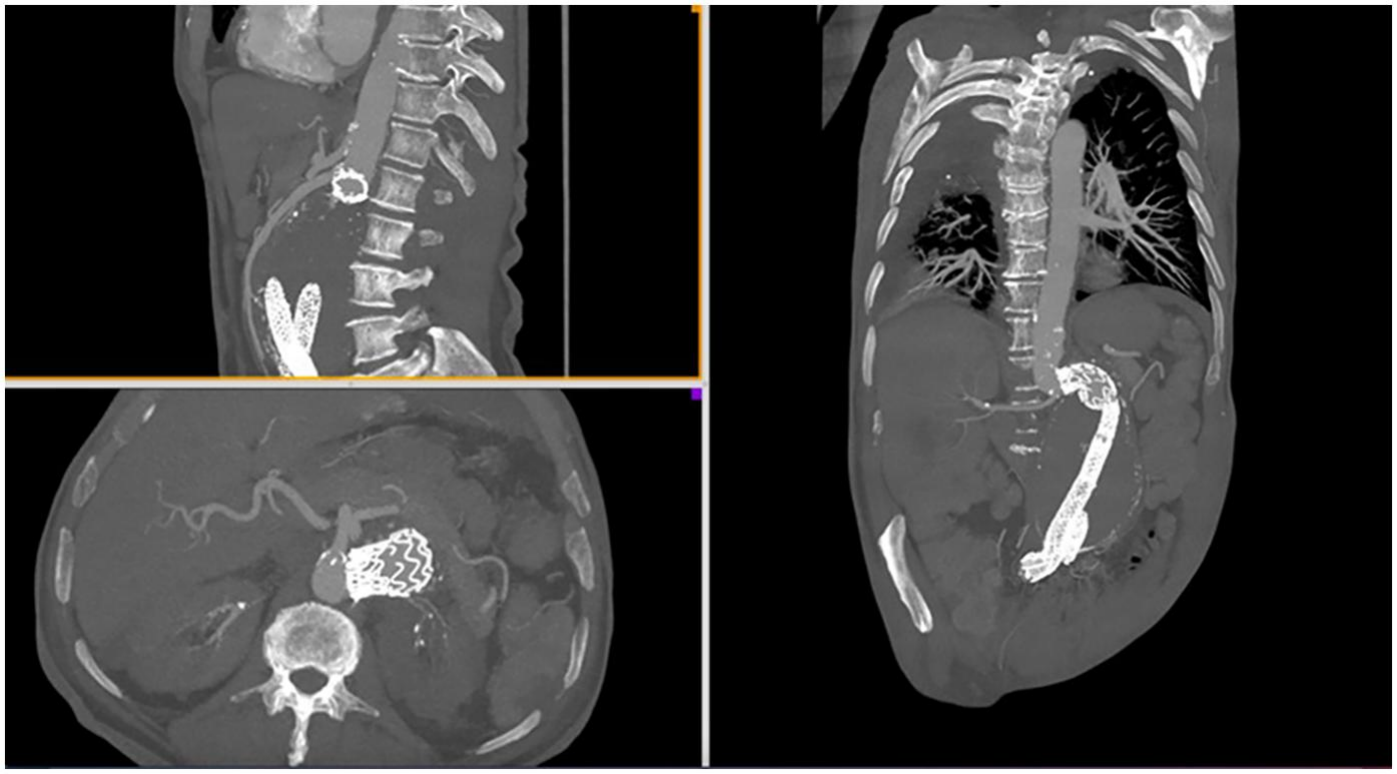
CT angiography imaging of a patient after graft implantation with aortic neck angulation of 110° and neck length of 10 mm.
